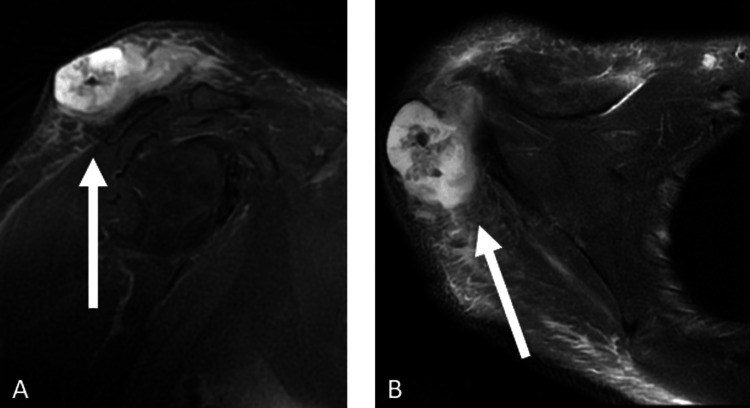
Preoperative magnetic resonance imaging (MRI) with intravenous (IV) contrast of the patient. (A) The pus collection in the sagittal plane is shown. (B) The pus collection in the axial plane is shown. White arrows indicate the abscess.
